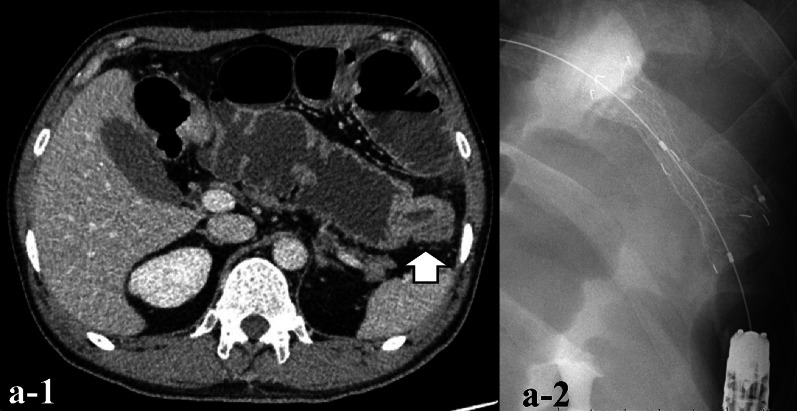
Abdominal CT and a colon stent. a-1 Contrast-enhanced peripheral wall enhancement (white arrow) was observed in the descending colon and splenic flexure. Dilation of the gastrointestinal tract was also observed. a-2 A colon stent was placed at the same site
